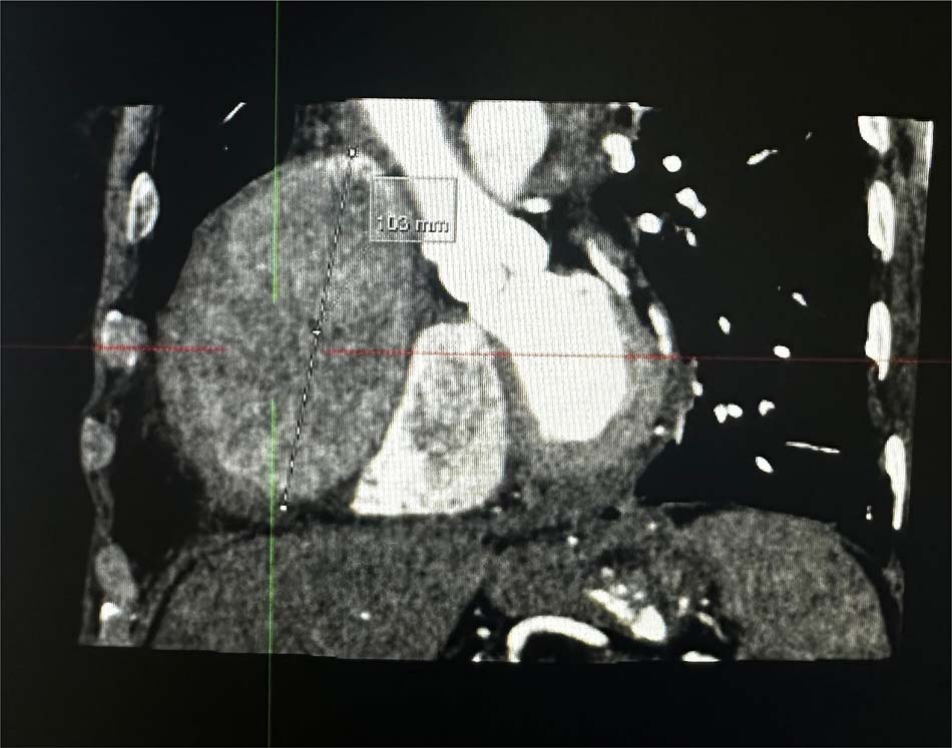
Coronal plane of a contrast thorax CT scan showing the giant coronary aneurysm.
